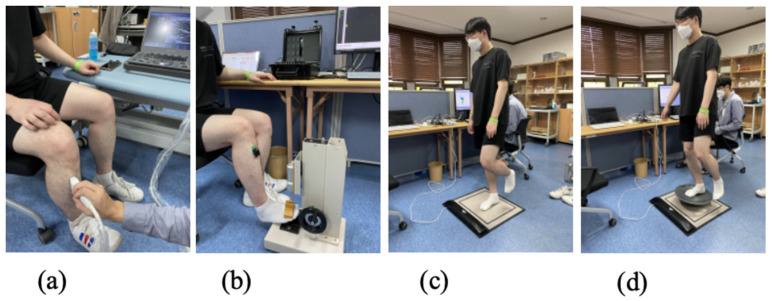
(a) Ultrasound imaging and measurements; (b) force and EMG measurements; (c) firm floor pressure sensor measurements; (d) balance board pressure sensor measurements.
